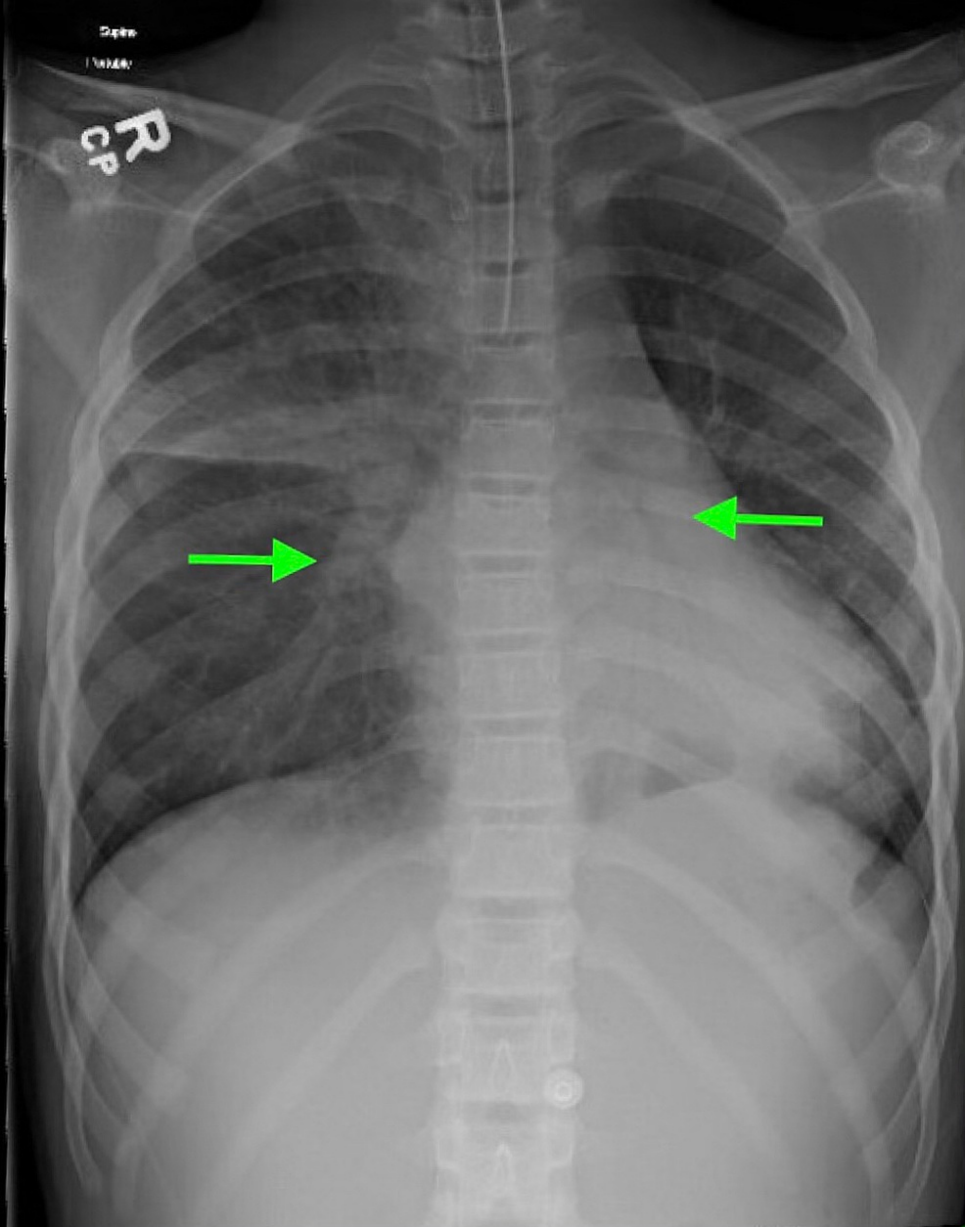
Chest X-ray of the patient on admission showing bilateral perihilar opacities (arrows)
